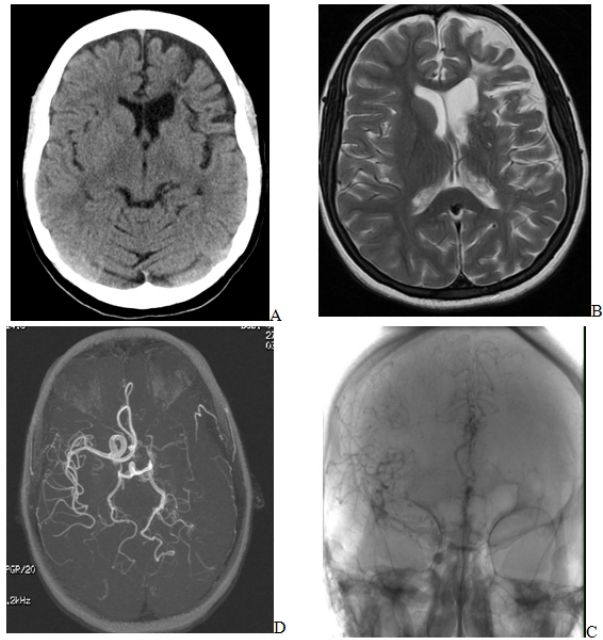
(A to D) with figure 1A showing ischemic changes in left frontoparietal region on CT scan brain plain, with figure 1B showing similar findings on T2 FLAIR MRI brain with enhancement. Figure 1C showing absence of arterial supply in the left MCA territory on MR angiogram while figure 1D showing absence of left internal carotid blood supply due to internal carotid occlusion.
